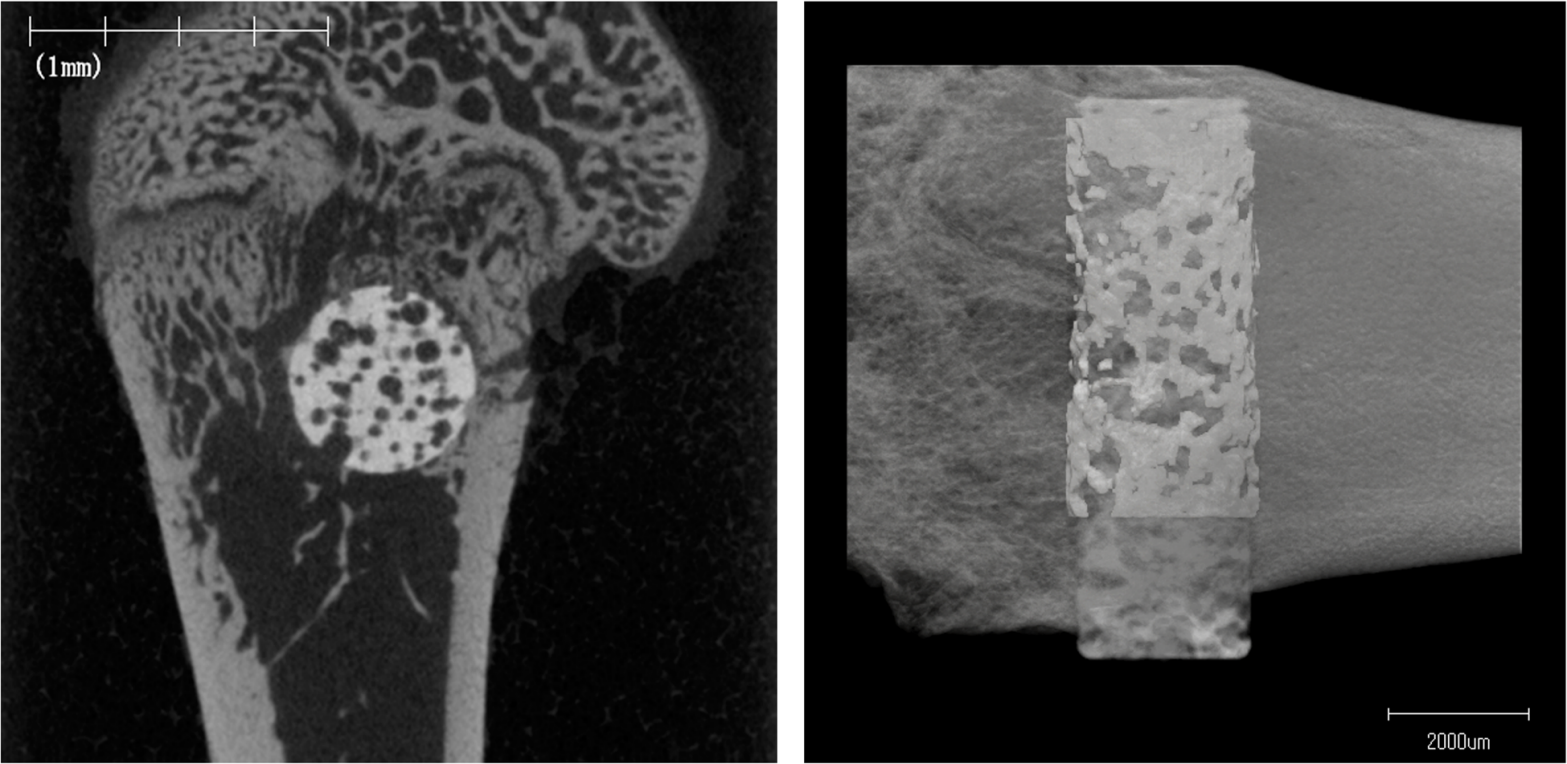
Microcomputed tomography. The right image shows a representative cross-sectional micro CT slice in the perpendicular to the longitudinal axis of the implant. The left image shows a representative three-dimensional computed tomography image around the implant
